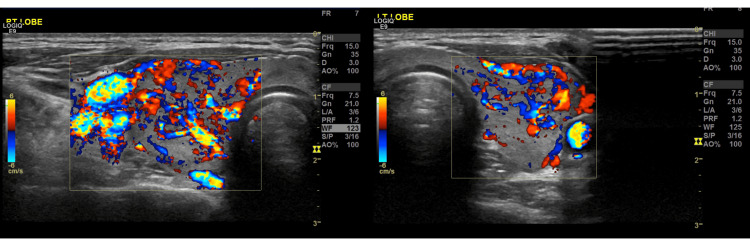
Thyroid ultrasonography showed bilateral increased vascularity.
